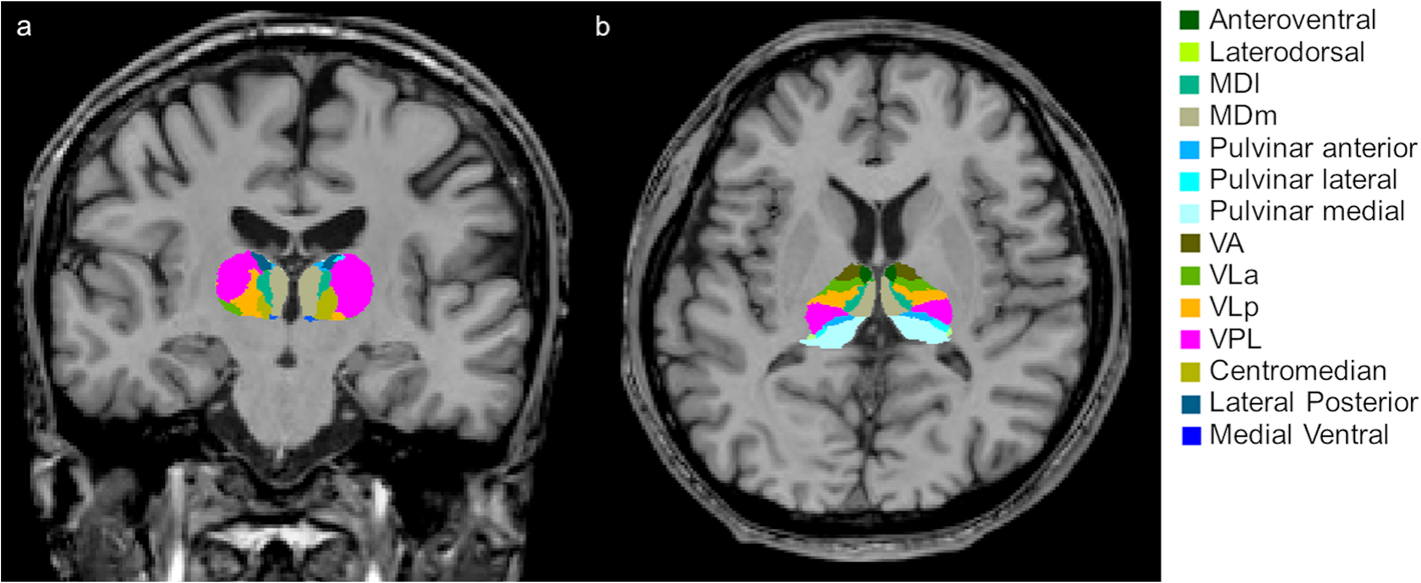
Example of segmentation of thalamic nuclei Segmentations and labels of thalamic nuclei in the coronal a and axial b plane generated by FreeSurfer (not all segmentations are shown). The segmentations are overlaid on the T1-weighted scan. MDl: mediodorsal lateral parvocellular, MDm: mediodorsal medial magnocellular, VLa: ventral lateral anterior, VLp: ventral lateral posterior, VPL: ventral posterolateral, VA: ventral anterior nucleus.
